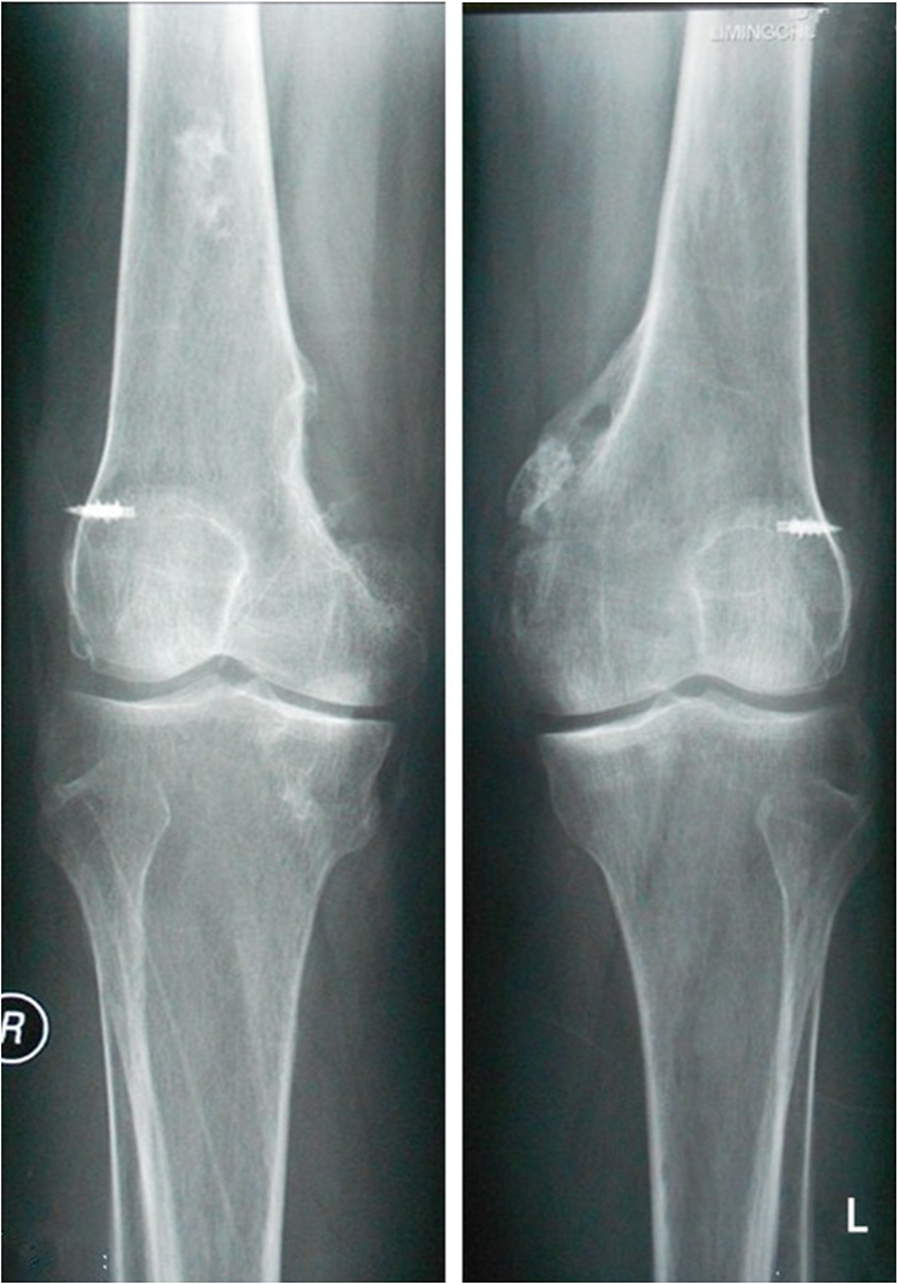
Postoperative radiographs of knees shows no loosening of rivets and no recurrence 18 moths after the excision of the ossific mass.
